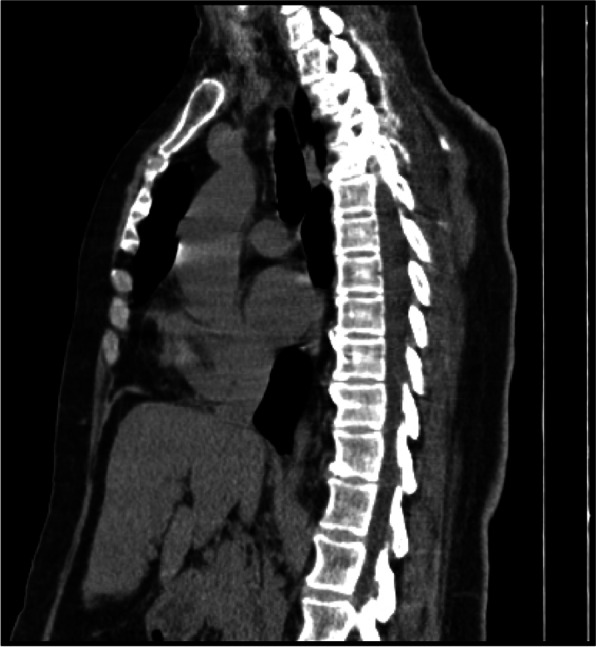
Sagittal CT scan demonstrating paravertebral spread of LA-dye mixture at T4-T5 (Right exit foramen)
